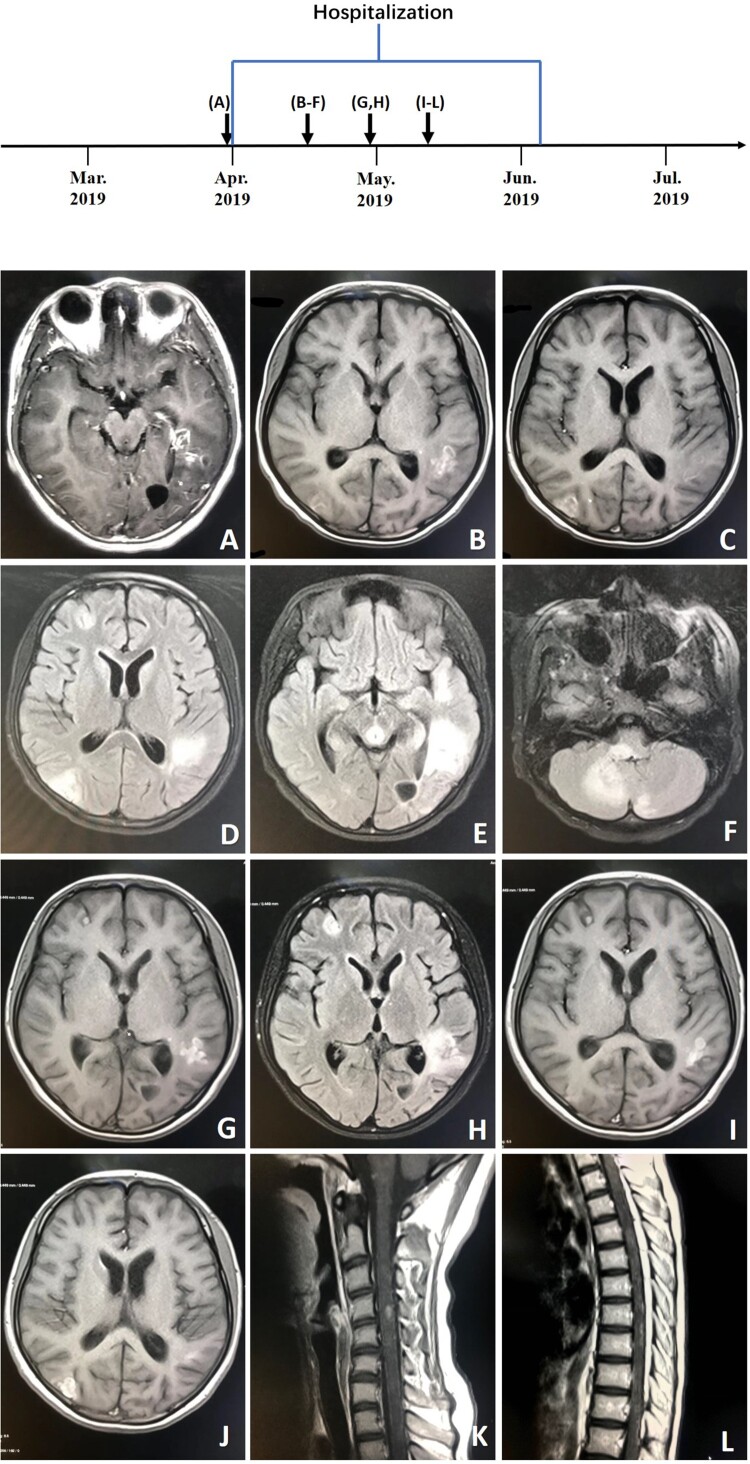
A, Axial T1-weighted enhanced image (March 28, 2019) showing multiple enhanced lesions throughout the brain, including the parietal lobe, left temporal lobe and occipital, and cerebellum. B, C, Axial T1-weighted images (April 13, 2019). D–F, Axial T2-weighted images (tirm dark fluid) (April 13, 2019) showing larger areas of multiple enhanced nodular lesions. G, Axial T1-weighted image (April 26, 2019). H, Axial T2-weighted image (tirm dark fluid) (April 26, 2019) showing new patchy lesions in the right frontal region. I–L, T1-weighted images (May 8, 2019) of the spinal cord and head showing new patchy lesions in the cervical and thoracic regions.
